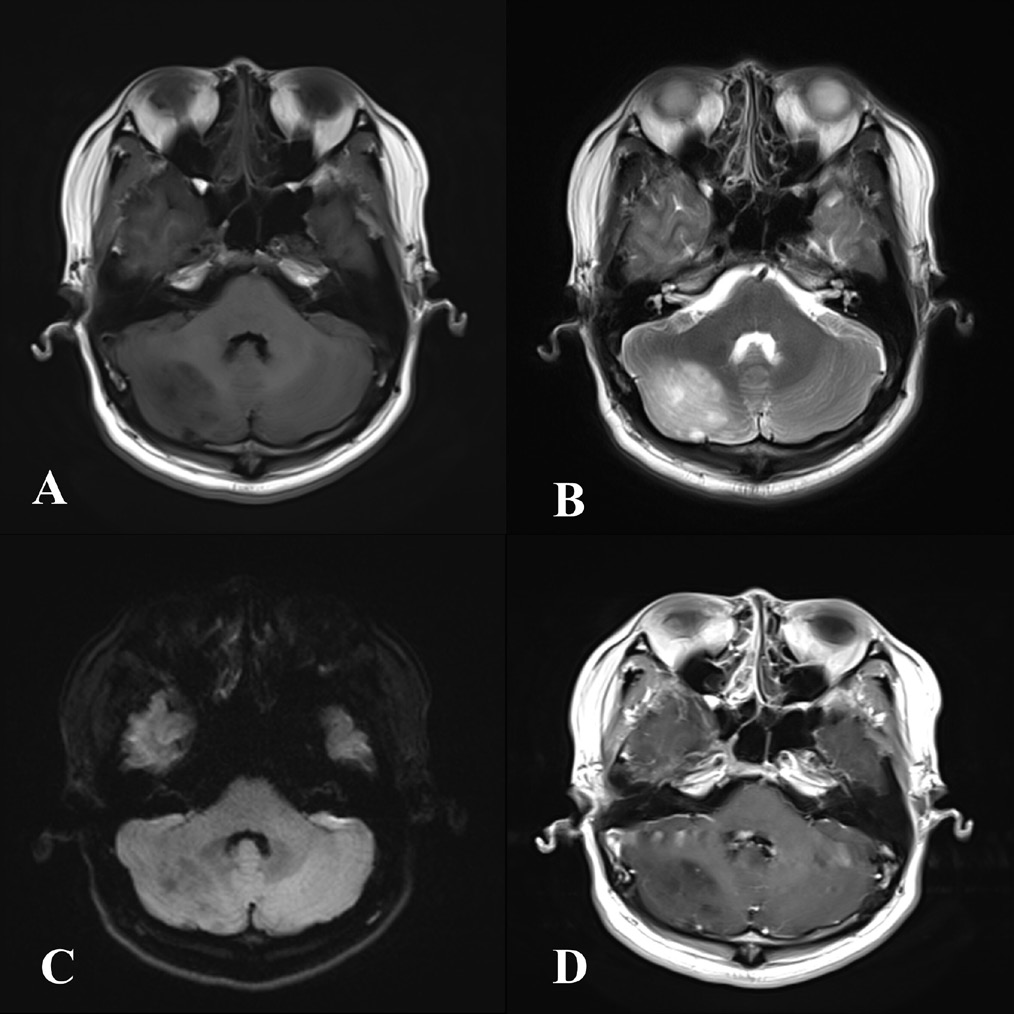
A 31-year-old male patient with no symptoms. MR examination: A. heterogeneous slightly hypointense signal on axial *T*_1_ weighted imaging; B. the lesion appears hyperintense on axial *T*_2_ weighted imaging, and the normal striation of the cerebellar hemisphere can be seen; C. hypointensity on DWI; D. no enhancement of the lesion was found on post-contrast *T*_1_ weighted MRI. DWI, diffusion-weighted imaging.
